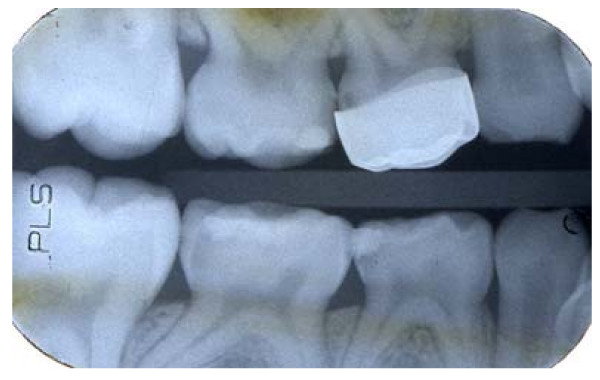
Radiograph of Hall PMC on tooth 54 (URD) recorded as unsatisfactory fit. Patient randomisation number 34.
